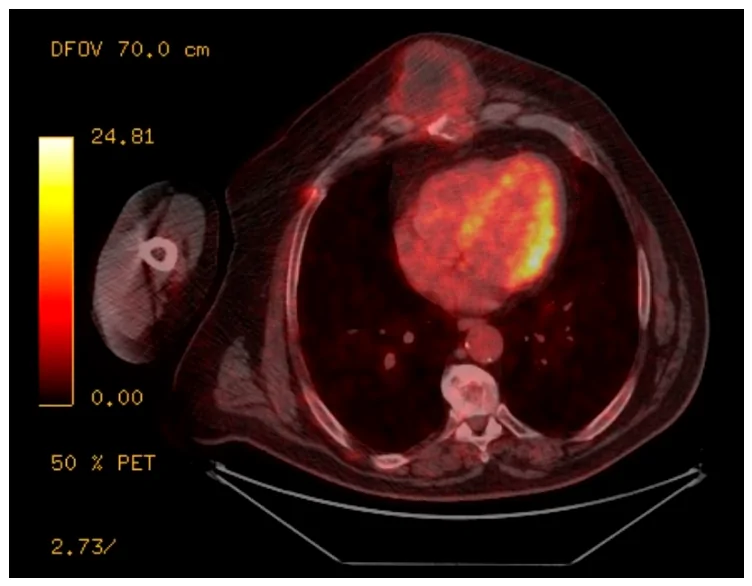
Preoperative axial fused PET/CT caption at the level of the lower heart and lower sternum.
